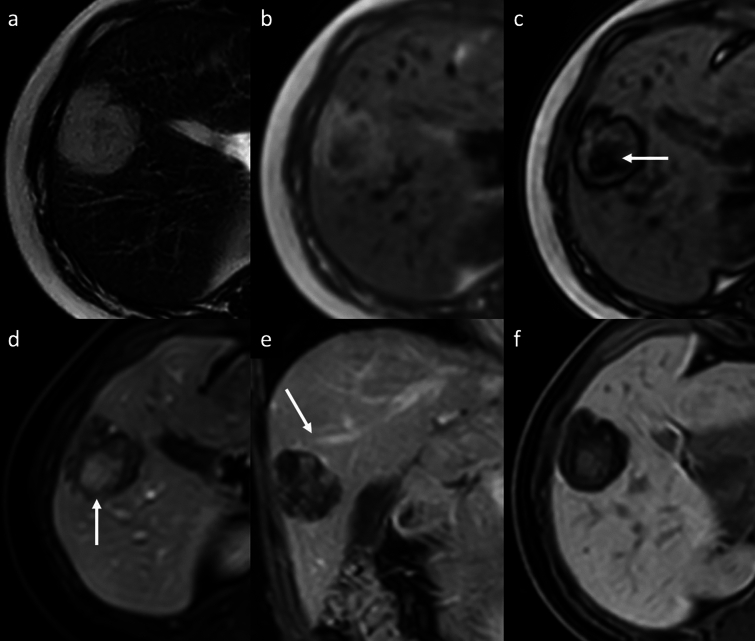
Hepatic angiomyolipoma in a 44-year-old woman. a Axial T2-weighted MRI shows a focal liver lesion arising in the right hepatic lobe with high signal intensity. Axial in-phase (b) and out-of-phase (b) gradient echo MR images show signal dropout at the center of the lesion, confirming the presence of fat in the lesion (arrow, c). Gadobenate dimeglumine-enhanced MRI obtained during the (d) arterial phase shows heterogenous low signal intensity of the lesion with small foci of peripheral discontinuous enhancement and a hypervascular central core (arrow, d). (e) Coronal T1-weighted image demonstrates the early venous drainage to the middle hepatic vein (arrow). The lesion shows a heterogenous appearance on (f) hepatobiliary phase acquired 2 h after the administration of contrast agent, displaying an hyperintense central core surrounded by a low signal intensity peripheral area
